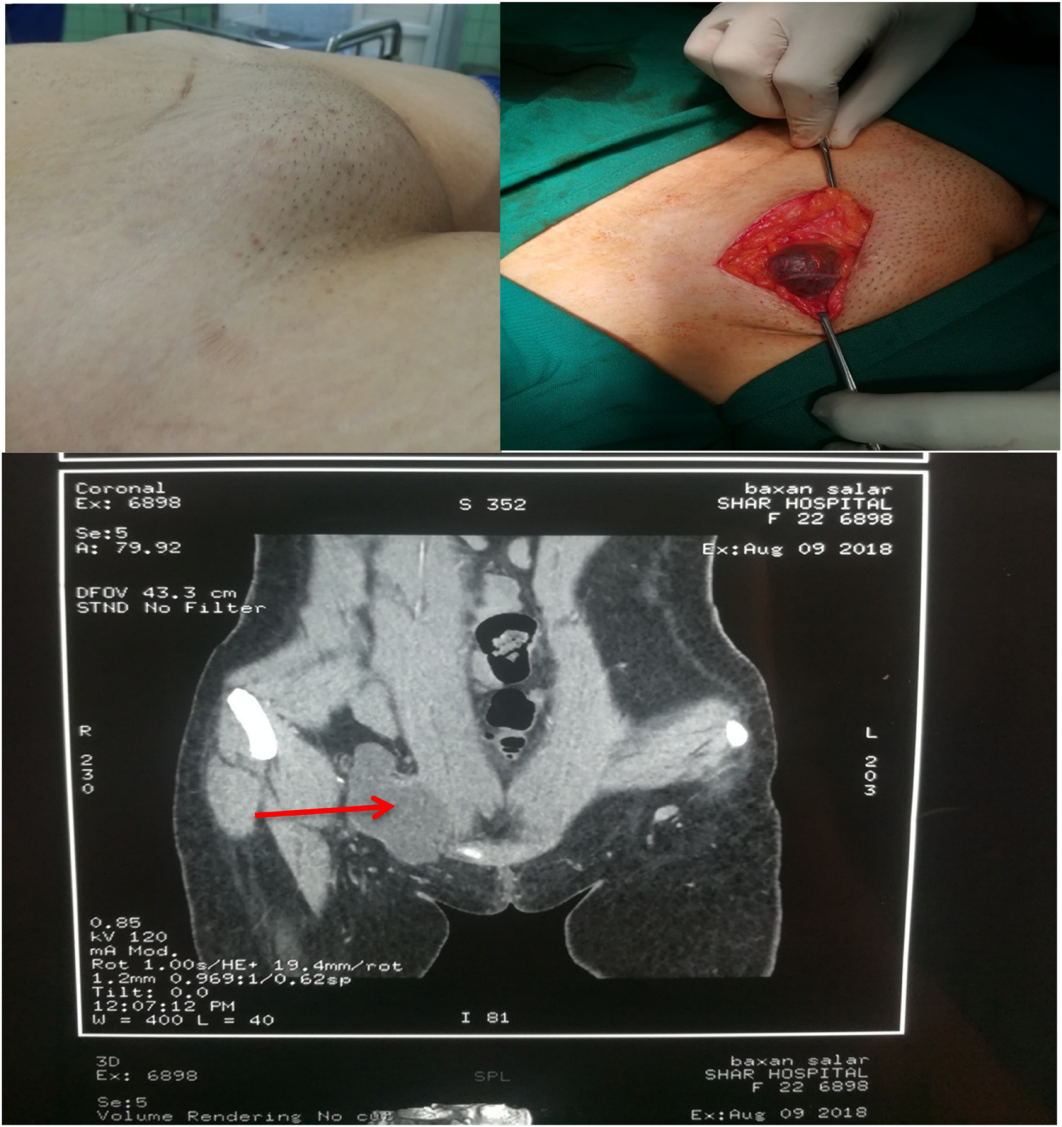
Coronal CT pelvis with IV contrast shows well well-defined lobulated cystic lesion in the right inguinal region characterized by a thin wall, no enhancing septa or solid component that was reported in patient number 2.
